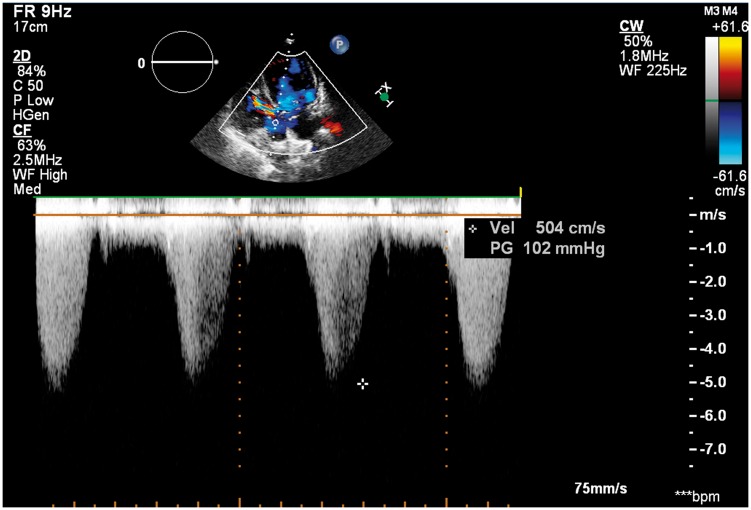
Apical five-chamber view: continuous wave Doppler examination showed the peak flow velocity across left ventricular outflow tract was 5.04 m/s.
